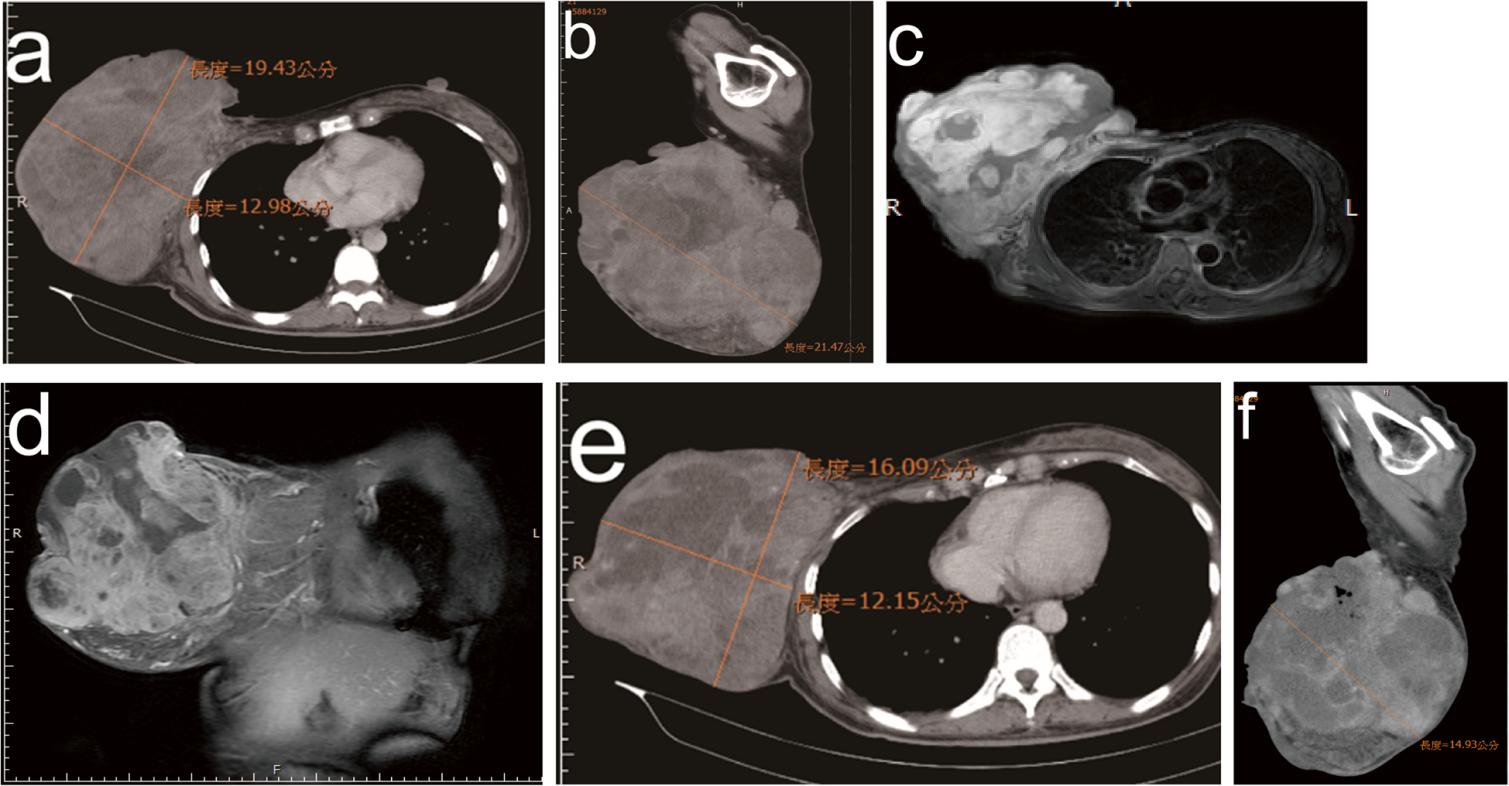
a and b Contrast-enhanced chest CT showed a huge lobulated heterogeneously enhancing mass (measuring approximately 19.43 × 12.98 × 21.47 cm) in the right breast. c and d Contrast-enhanced MRI showed a 20-cm lobulated right breast mass with right major pectoralis muscle invasion. e and f. After four courses of TACE, follow-up CT revealed tumor reduction of 45% of total volume (measuring approximately 16.0 × 12.0 × 15.0 cm)
